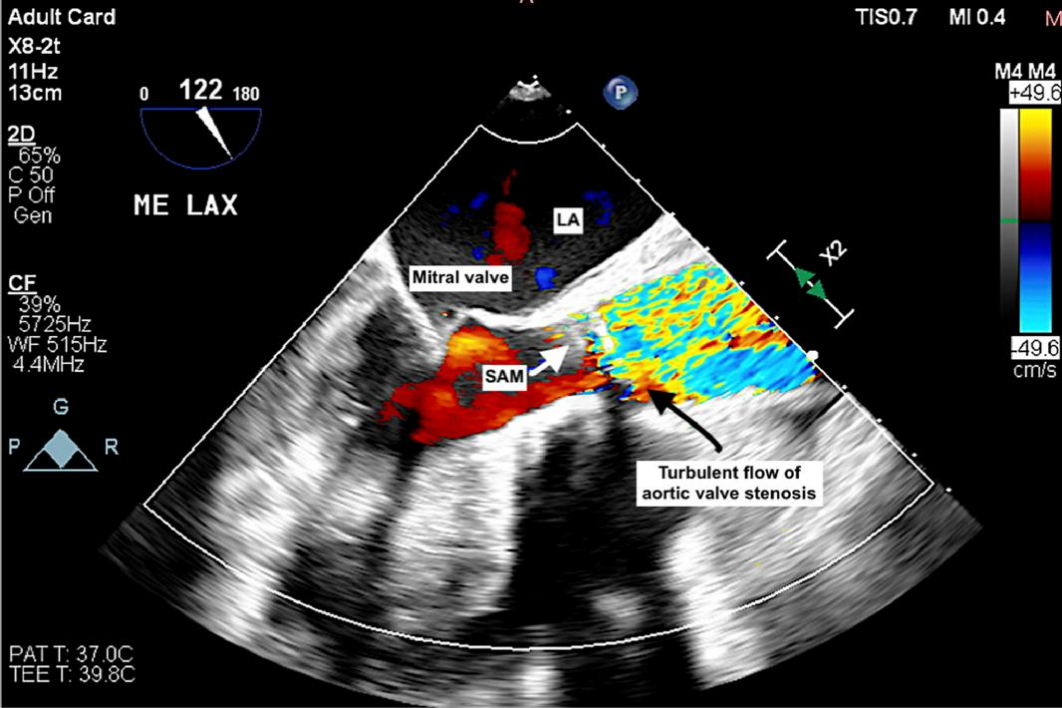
Transoesophageal echocardiography with colour Doppler demonstrating the presence of a turbulent blood flow through the aortic valve.
